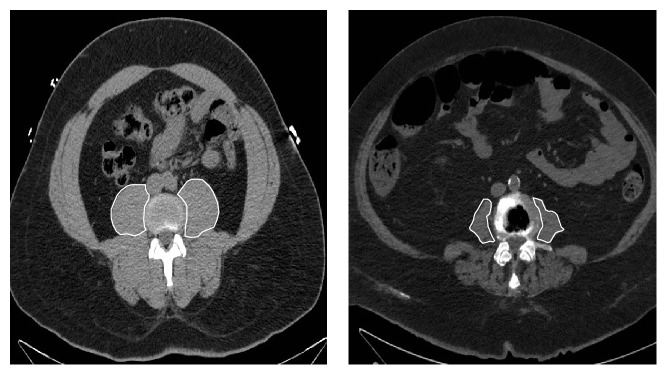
Example calculation method for TPA based on axial CT scan at L3 in two patients. Notice the difference in TPA between them.
